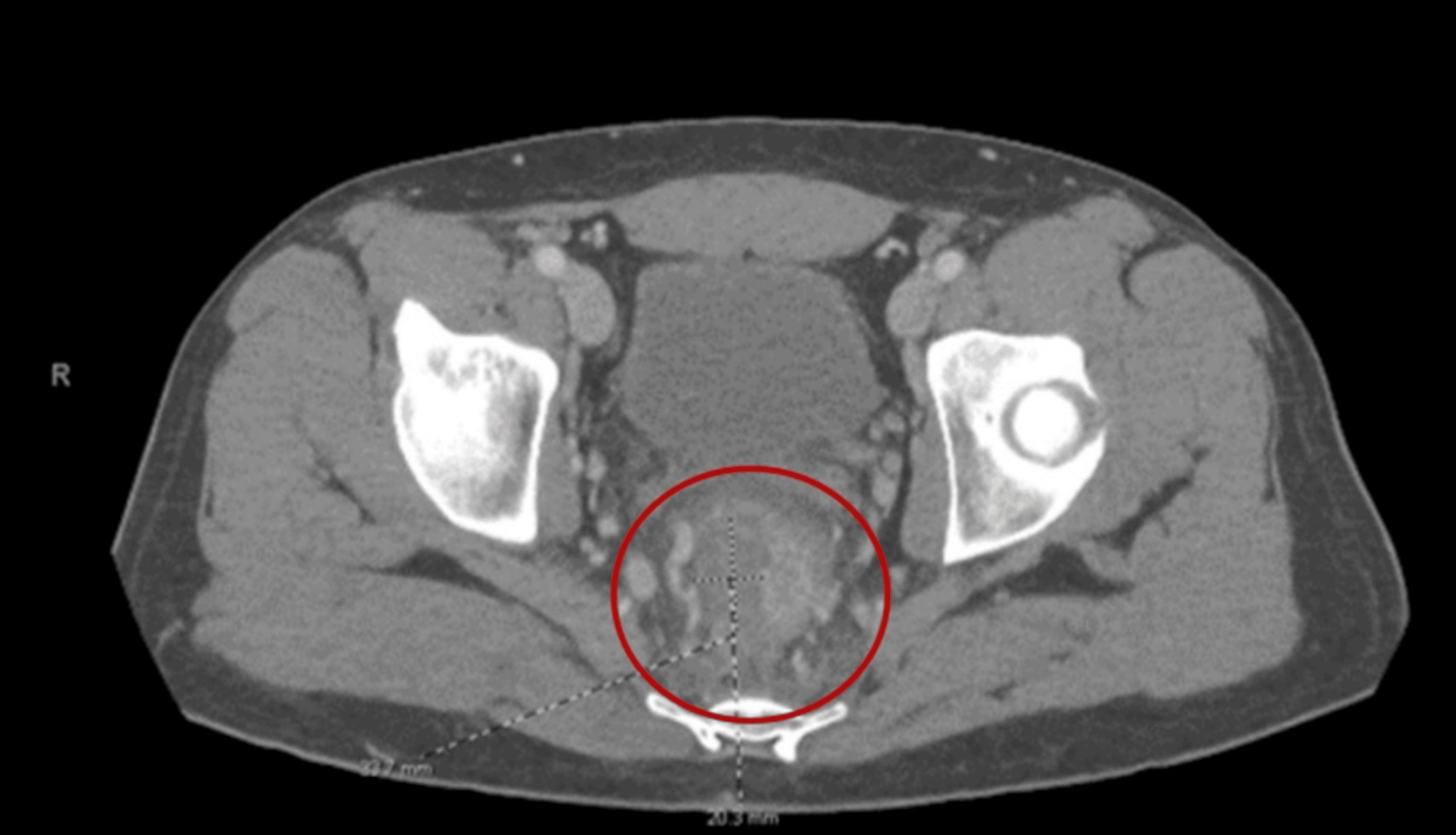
CT scan of the distal rectum showing a short segment with asymmetric wall thickening, measuring up to 2 cm in thickness.
